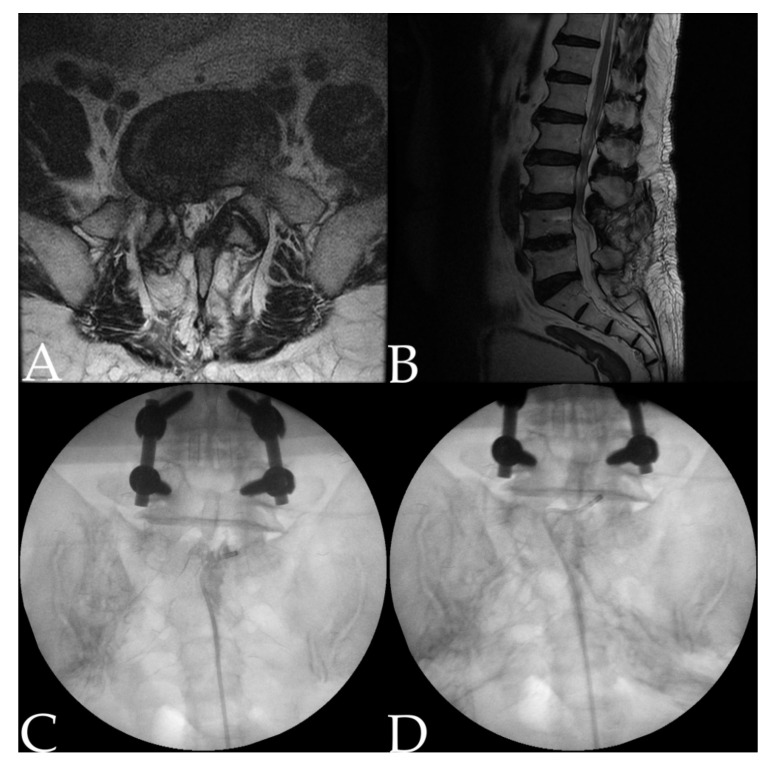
A 59-year-old man underwent L4–5 fusion surgery five years ago for excruciating pain from spinal stenosis. Afterward, the pain improved, and then bilteral leg pain began. He underwent adhesiolysis one year after the onset of symptoms. (A) Axial magnetic resonance image (MRI) showed the L5–S1 level with suspected adhesion around the central canal. (B) Sagittal MRI showed no specific abnormality at the L5–S1 level. (C) Epidurogram confirmed filling defect at the central canal of the L5–S1 level. (D) Contrast flow was observed at the L5 vertebra body level after mechanical adhesiolysis with catheter.
